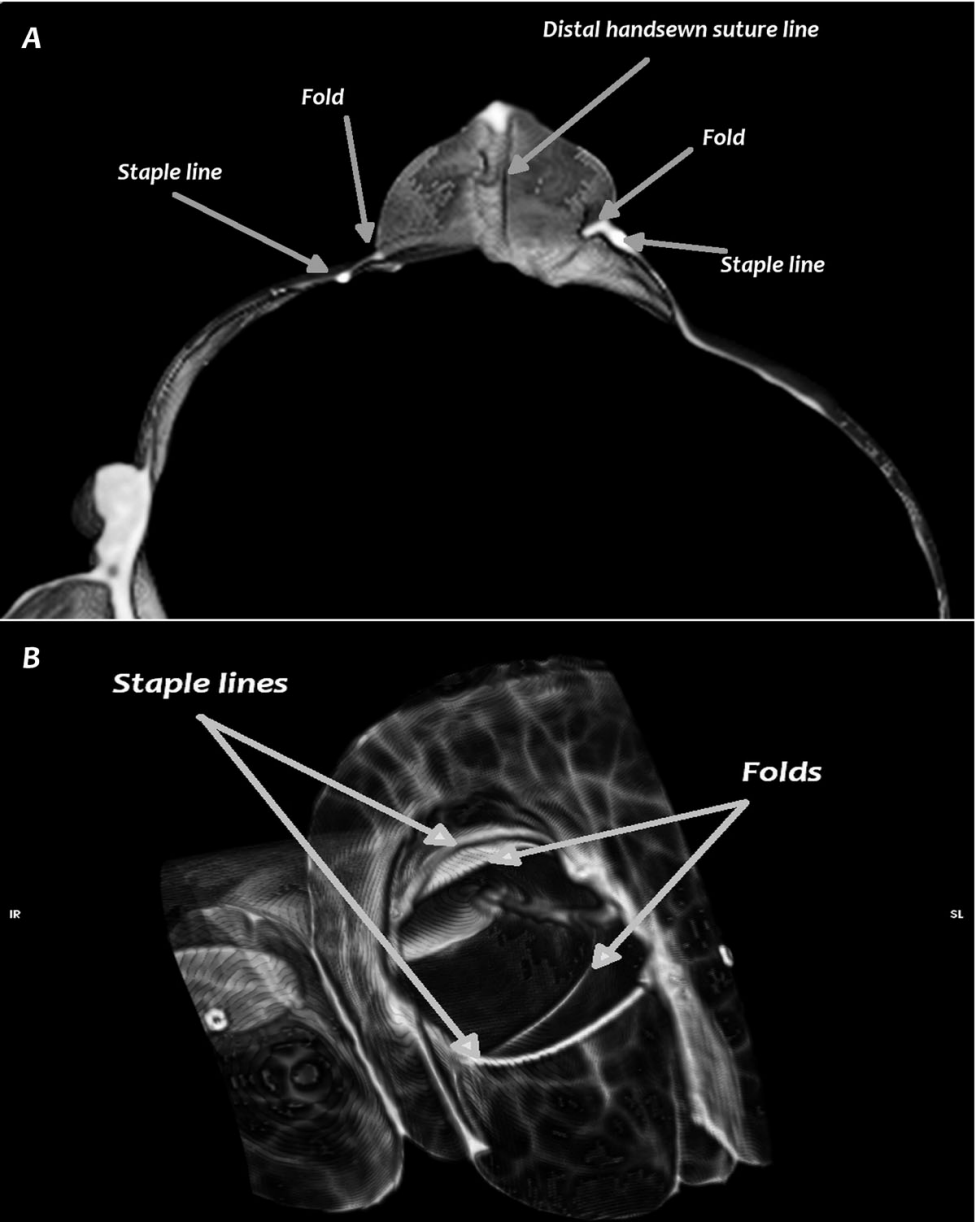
CT scan of the Freeman technique. Note the two folds of tissue in the jejunal wall inside the stoma, not corresponding to staple lines. A) transverse view, B) 3D reconstruction of the anastomosis, viewed from inside the cecum.
